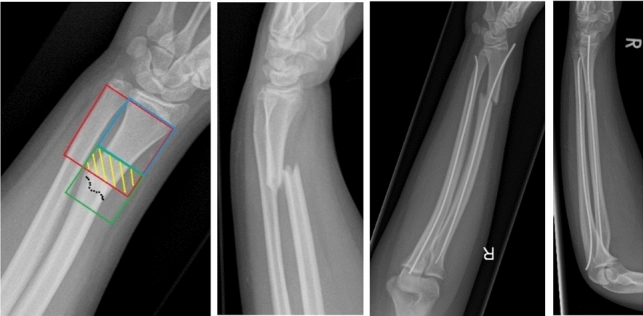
a–d. a, b a.p. and lateral radiograph of a diametaphyseal radius fracture in a 14-year-old girl. The black dotted line marks the fracture line. The red square marks the metaphysis, the yellow area marks the diametaphyseal junction zone (DMJZ) as defined by Lieber et al., the green square marks the DMJZ defined as the area of the radius with a forearm fracture index between 1 and 2. c, d postoperative a.p. and lateral radiographs after reduction and osteosynthesis with elastic stable intramedullary nailing (ESIN). The distal radius fragment presents with moderate translation and ulnar deviation in the a.p. radiograph
